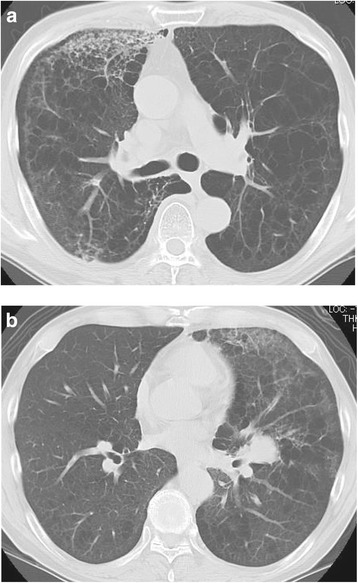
Computed tomography images of nivolumab-induced pneumonitis in Case 3. a Non-segmental ground-glass opacities and consolidations were observed in a predominantly subpleural distribution at both lungs on the 14th day of the initial nivolumab treatment. b After the predominant lesion at the right lung in the initial disease episode was reduced, the similar opacity was newly observed at the left lung in the time of relapse
